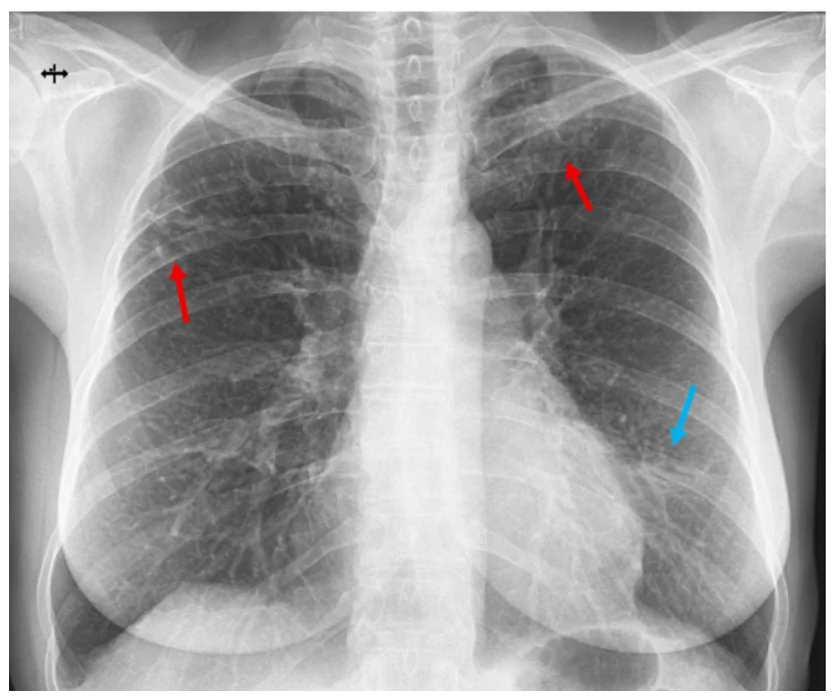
Chest X-ray posteroanterior view showing reticular and reticulonodular opacities of moderate intensity, partially confluent in areas, predominantly distributed in the upper third of both lung fields, more pronounced on the right side (red arrows). A moderate-intensity opacity with irregular margins is also observed adjacent to the cardiac silhouette (blue arrow).
